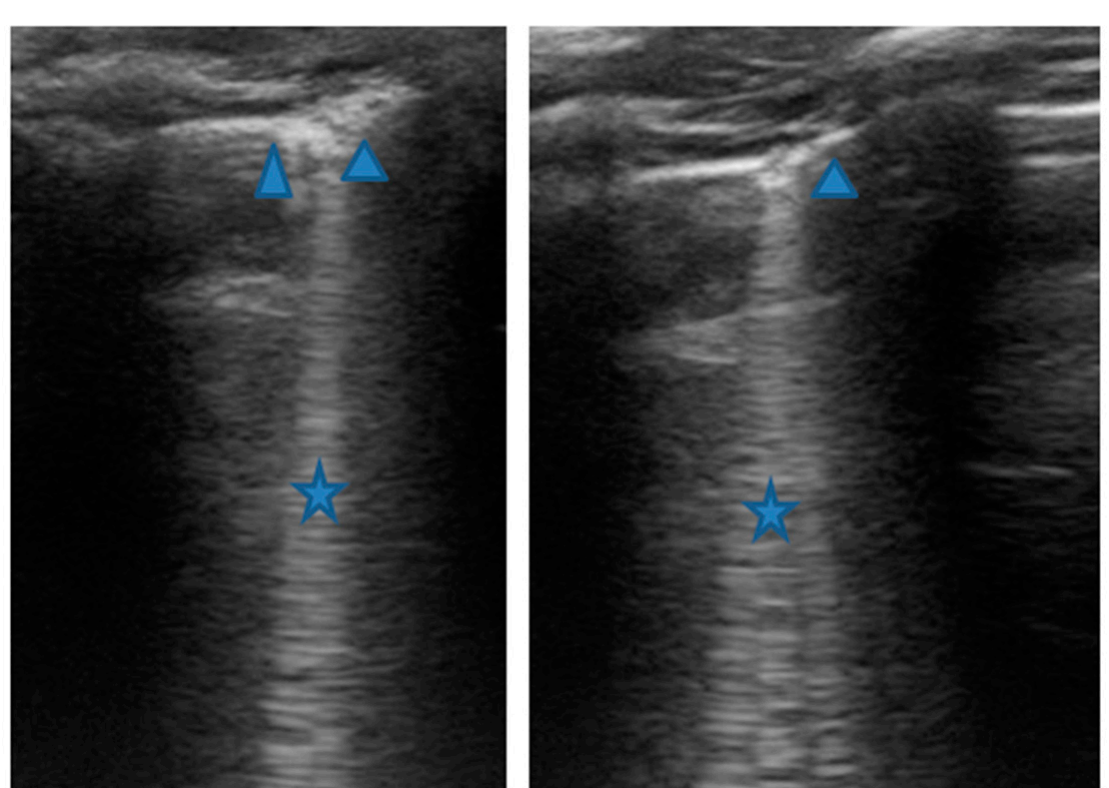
Grayscale lung ultrasound examination shows: sonographic interstitial syndrome (SIS), characterized by long confluent vertical artifacts (asterisks), associated with an irregular pleural line, and distributed over all the explored fields in an inhomogeneous way.
